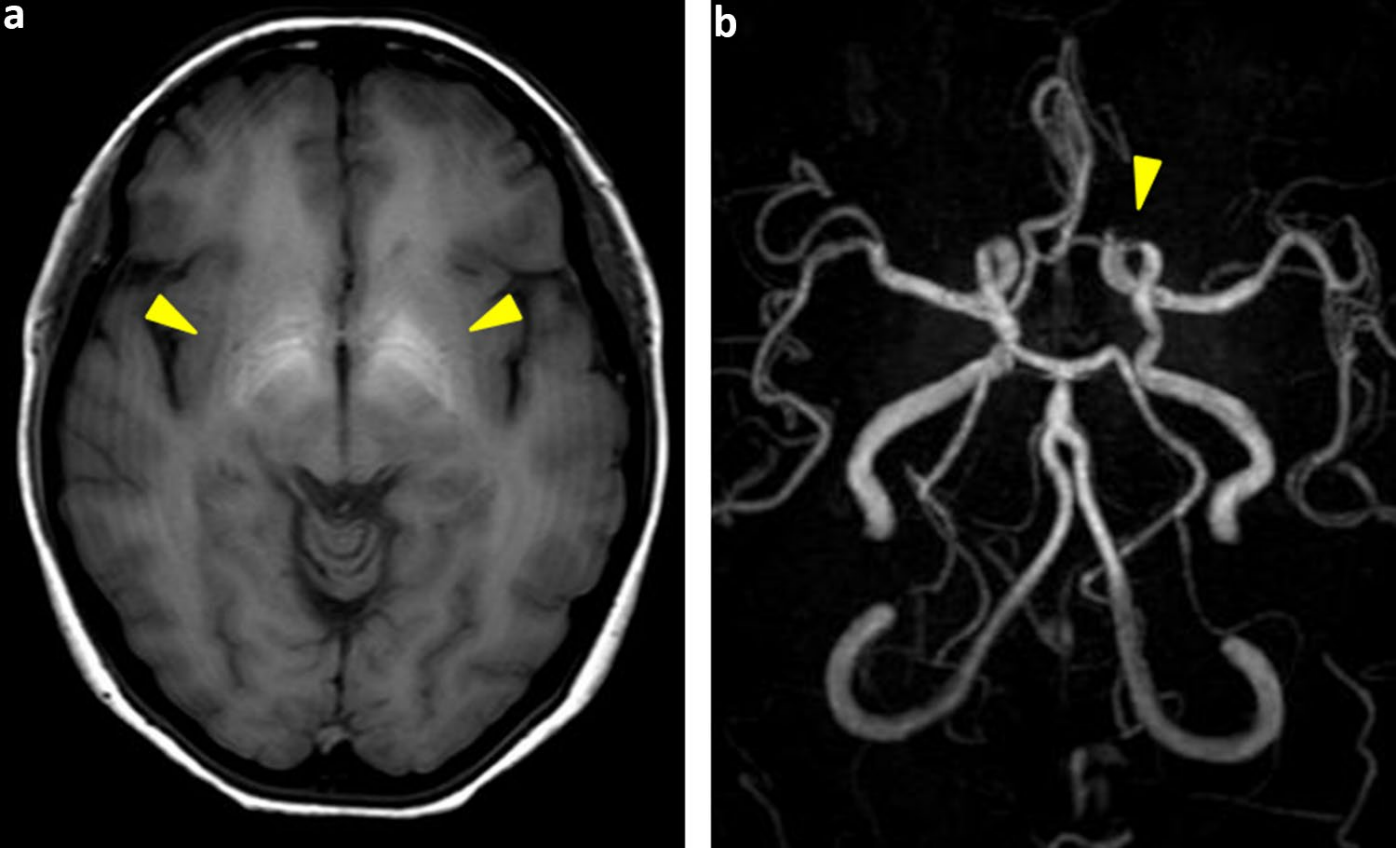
Head MRA examination (June 20XX + 3) a High-signal intensity is observed in the bilateral globus pallidus on T1-weighted imaging. b MRA shows decreased signal intensity in the left internal carotid artery (C3), suggesting the possibility of stenosis. MRA magnetic resonance angiography
